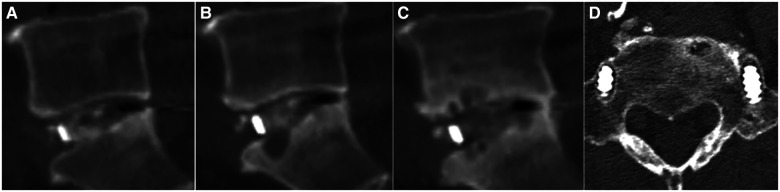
A 69-year-old Male patient with lumbar spinal stenosis underwent cage implantation. (A) Sagittal lumbar CT scan performed at postoperative 3 days showed good bone filling in the inner cage zone and no bone graft in the posterior cage zone. (B) CT scan performed at postoperative 3 months showed cage subsidence and educed upper and lower endplate space in the posterior zone. (C) CT scan performed at postoperative 2 years showed a lucent line without bone remodelling or passage of the bone trabecula in the inner cage zone. However, complete bone remodelling and passage of the bone trabecula in the posterior cage zone were observed. (D) Cross-sectional lumbar CT scan performed at postoperative 2 years showed loosening of the pedicle screws.
